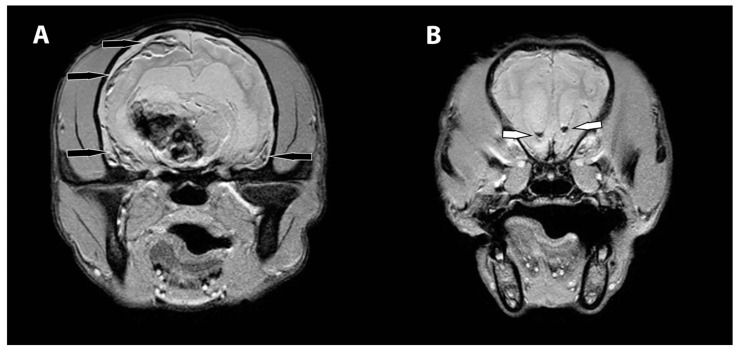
MRI images of the subdural and intraventricular hemorrhage. The transverse T2* weighted images (A) display heterogenous, mostly hypointense band of hemorrhage in the region of the subdural space, right worse than left (black arrows). More rostrally, the transverse T2* weighted images (B) show foci of signal void within the lateral ventricles, consistent with intraventricular hemorrhage (white arrows).
